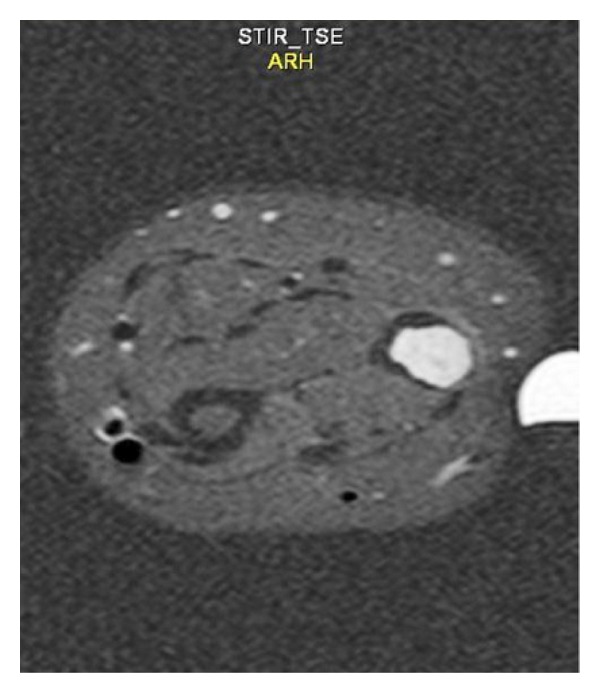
Fat-saturated fast spin-echo T2-weighted axial image showed a well-defined high signal ovoid intramedullary lesion measured 2*1 cm in the distal diaphysis of left ulnar bone that was protruding exophytically from medial surface of ulna bone.
